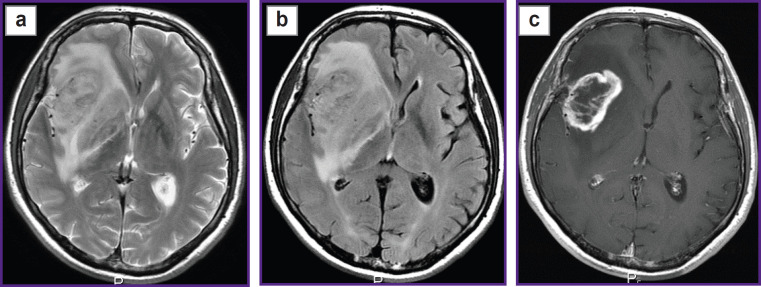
Patient with glioblastoma in the right fronto-insular region: (а) Т2-weighted MRI, (b) Т2-FLAIR images, (c) contrast-enhanced Т1
